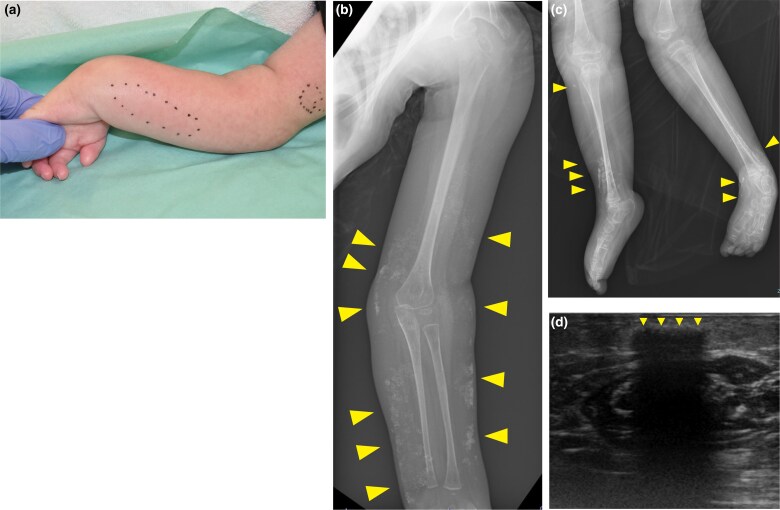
(a) Nontender subcutaneous nodules in the left arm. No significant changes in the epidermis were observed. (b, c) Radiography revealed extensive subcutaneous nodules (arrowhead) in the extremities. (b) Left arm. (c) Lower limb. (d) High-frequency ultrasonography showed hypoechogenic masses with posterior acoustic shadows (arrowhead).
